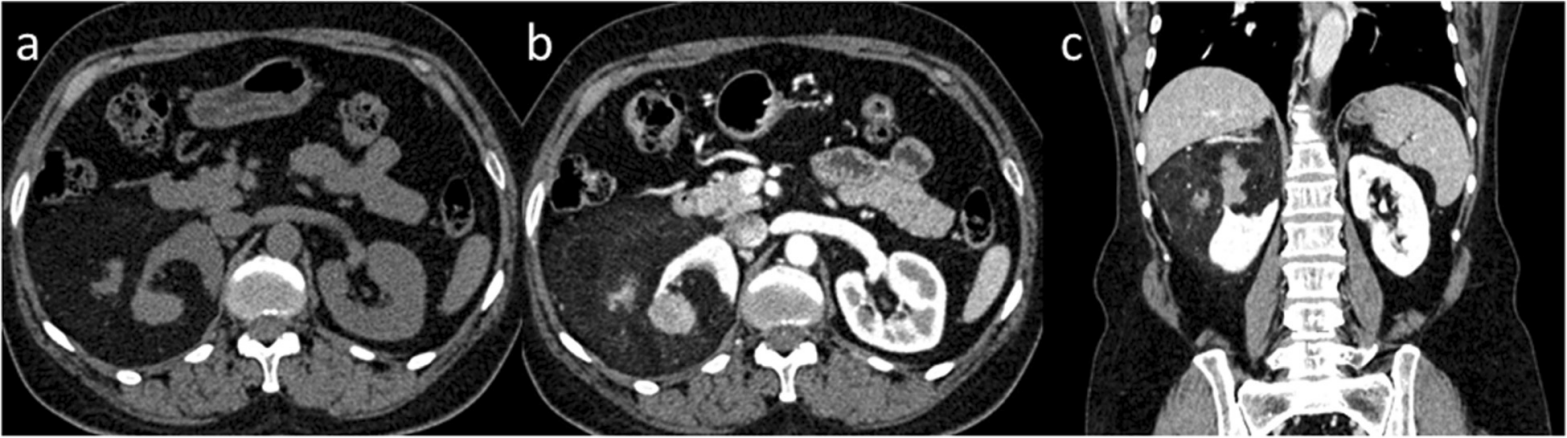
Macroscopic fat containing E-AML in a 62-year-old woman. Non-contrast axial CT image (a) demonstrates a slight hyper-density mass in the right kidney with abundance of fat and large amounts of soft tissue. Contrast-enhanced CT image of venous phase (b) and coronal reconstruction image of delayed-phase (c) demonstrate heterogeneous enhancement
